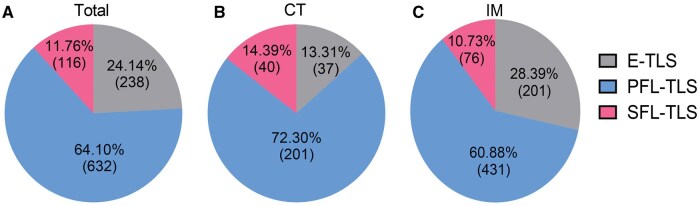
Characteristics of TLS maturation subtype spatial distribution in pMMR RC. Pie charts depict the distribution and proportions of TLS subtypes at different maturation stages in various tumor regions. The distribution and proportions of E-TLSs, PFL-TLSs, and SFL-TLSs in (A) total tumor region, (B) CT, and (C) IM.
